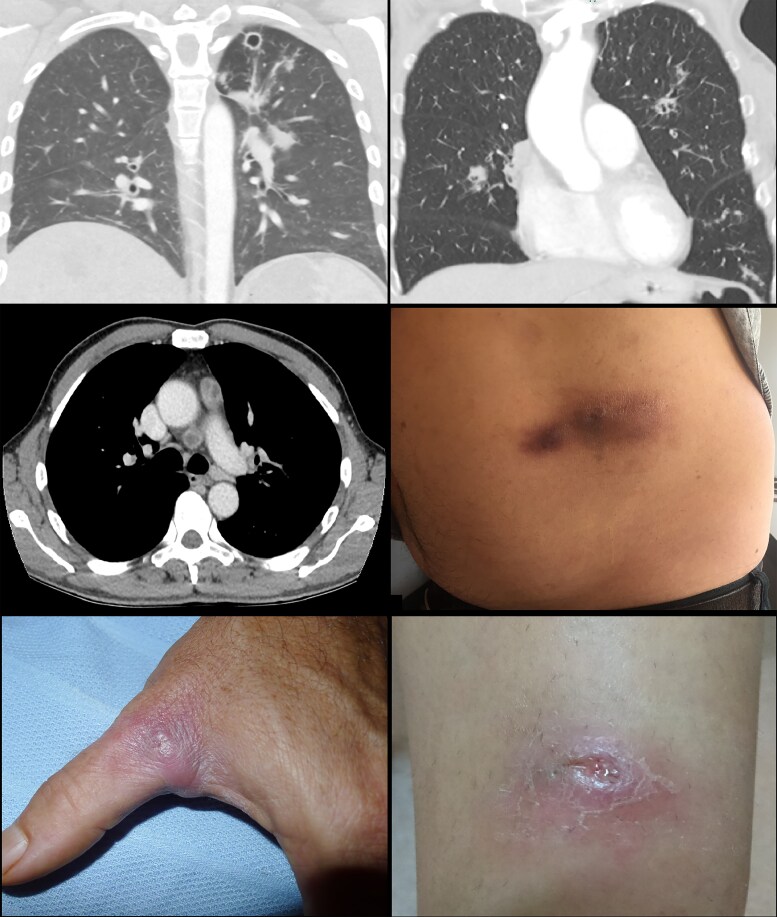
Typical clinical findings in chronic melioidosis. Top left and right: computed tomography (CT) images of cavitating pulmonary lesions. Middle left: CT image of mediastinal lymphadenopathy. Middle right, bottom left and right: cutaneous nodules and ulcer.
